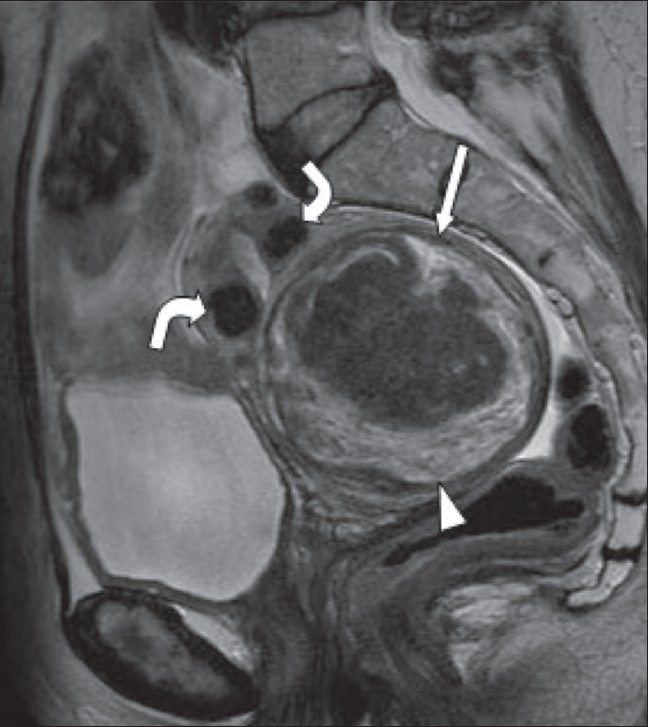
A 71-year-old woman shown to have uterine fibroids whilst undergoing an MRI scan of her hip. She had no gynecological symptoms. Sagittal T2W MRI image shows a 7-cm intramural fibroid (arrow), with surrounding high signal (arrowhead). Several other small intramural fibroids (curved arrows) and a trace of fluid in the pouch of Douglas are also present
